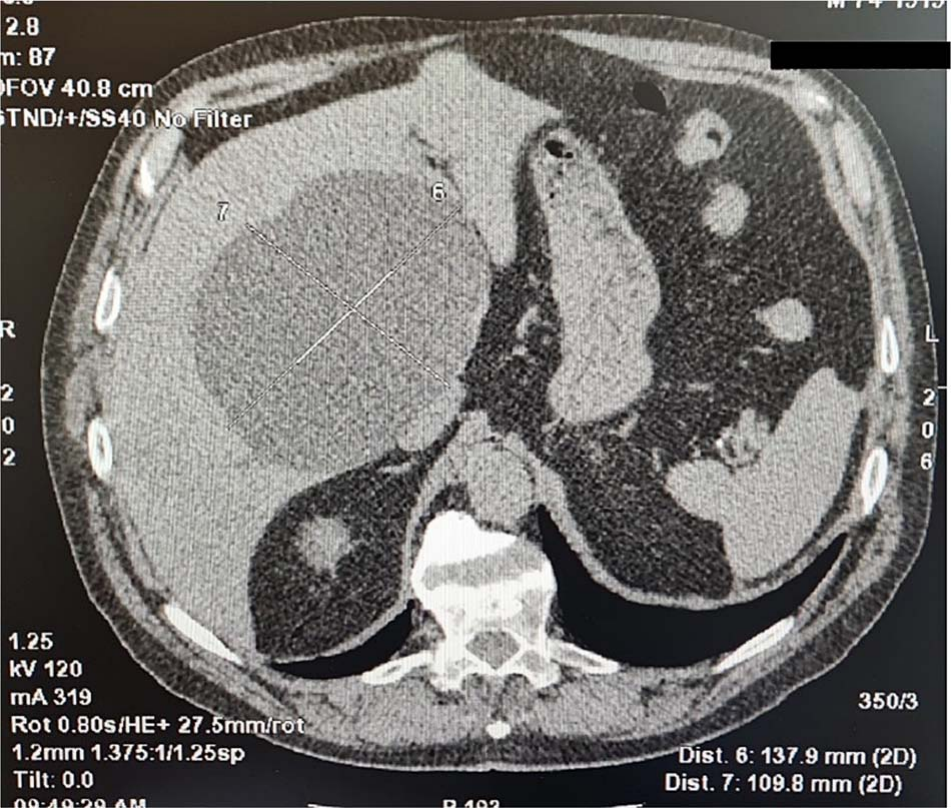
Computed tomography imaging of the liver cyst.
